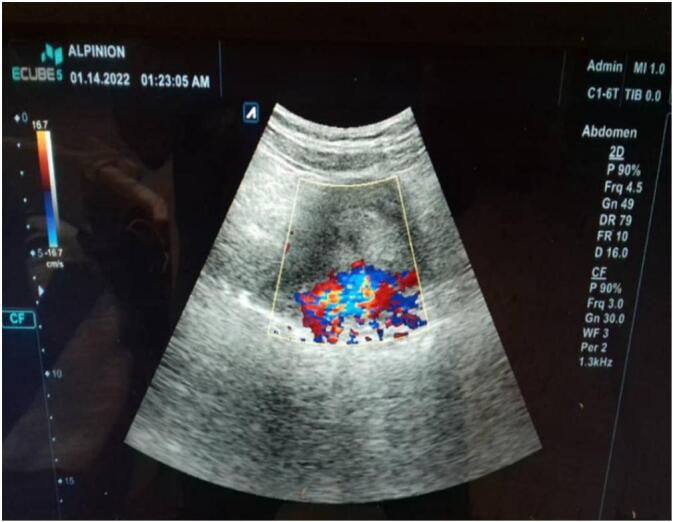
Trans Vaginal Sonography with Doppler showing an AVM mass behind the uterus.
